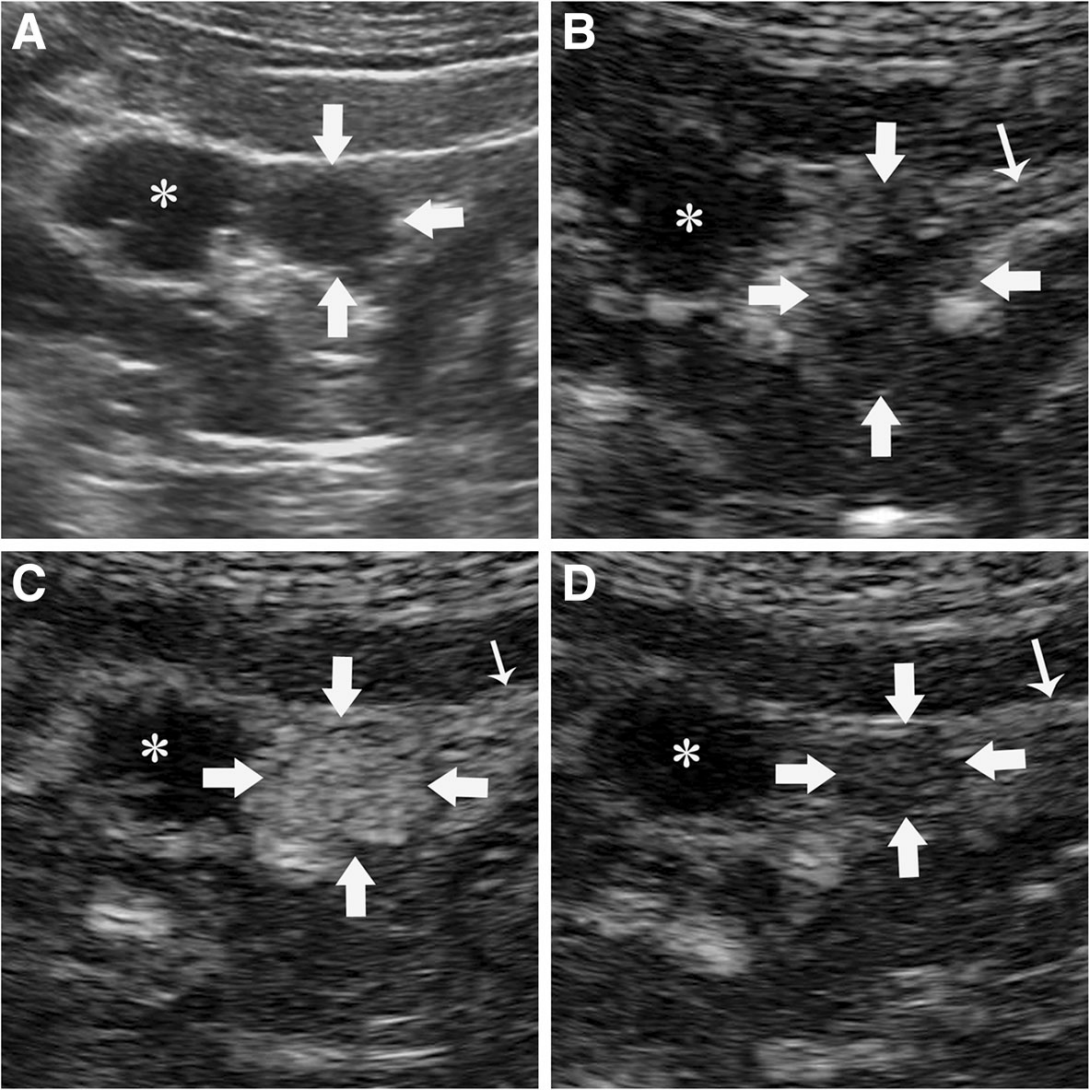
The contrast-enhanced ultrasonography features of the tumor in native left kidney .a the tumor (arrow) and cysts (asterisk) are presenting hypoechoic lesions in conventional ultrasonography which is difficult to differentiate from surrounding cysts. b. After injection of contrast agent, the edge of tumor (wide arrow) start enhancing at 20 s, showing hypo-enhancement as surrounding parenchyma. c The lesion achieved peak-enhancement at 33 s, more intense than surrounding kidney parenchyma (narrow arrow). d The lesion gradually turned to hypo-enhancement at 90 s, showing hypo-enhancement in comparison to surrounding parenchyma .The cyst (asterisk) around the lesion still non-enhancing during whole examination
